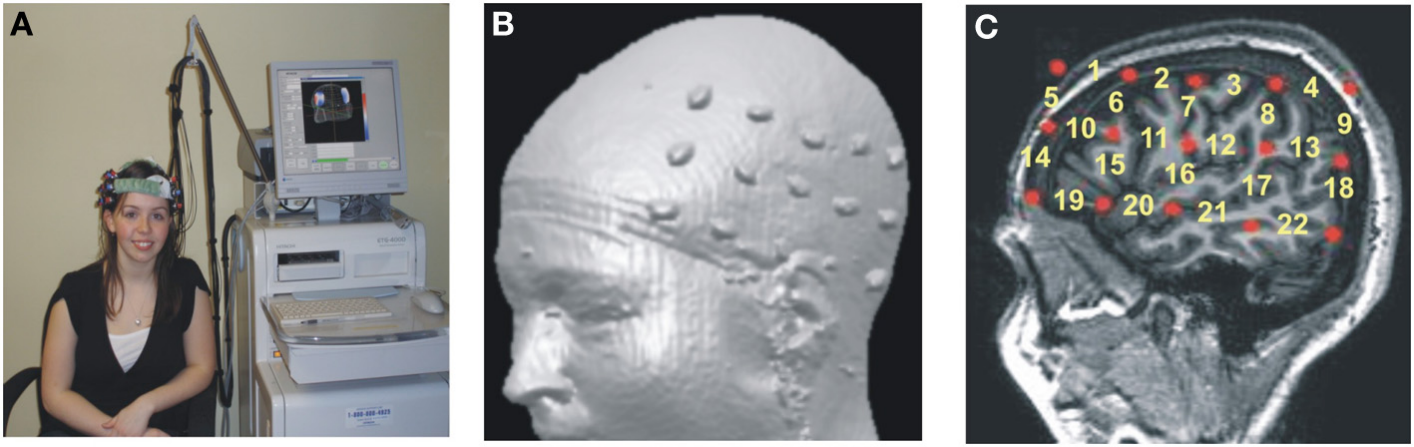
Hitachi ETG-4000 Imaging System MRI Neuroanatomical Co-Registration. (A) Participant with a Hitachi 48-channel ETG-4000. Optodes in place and ready for data acquisition. The 3 × 5 optode arrays were positioned on participants' heads using rigorous anatomical localization measures including 10 × 20 system and MRI coregistration. (B) MRI co-registration was conducted by having nine healthy adults wear two 3 × 5 arrays with vitamin-E capsules in place of the optodes in MRI. (C) Anatomical MRI images were used to identify the location of optodes (Vitamin E capsules) with respect to underlying brain structures (sagittal section shown here). Location of the fNIRS channels numbered in yellow.
